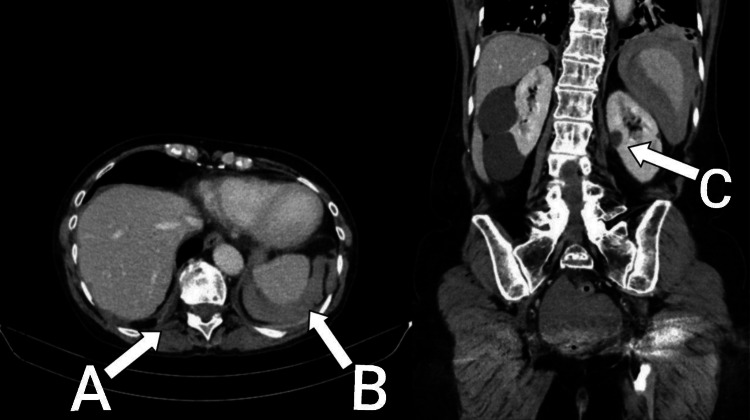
CT of the abdomen. Moderate-volume free fluid is present within the pelvis and upper abdomen, surrounding the liver and spleen. Around the liver, the fluid has a mean attenuation of approximately 55 Hounsfield units, suggesting complex rather than simple fluid (arrow A). The majority of high-attenuation free fluid, which could represent blood, surrounds the spleen in the left upper quadrant (arrow B), raising the possibility of hemoperitoneum secondary to splenic rupture. There is no evidence of free air beneath the right or left hemidiaphragm. Bilateral renal cysts are noted (arrow C), with no suspicious renal mass or hydronephrosis. Bilateral hip prostheses degrade image quality in the pelvis. There is no evidence of small or large bowel obstruction.
